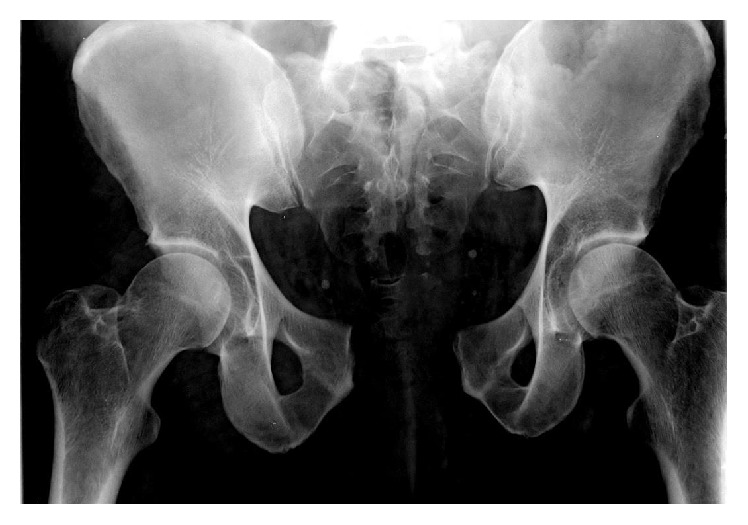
Presenting pelvis injury X-ray.
